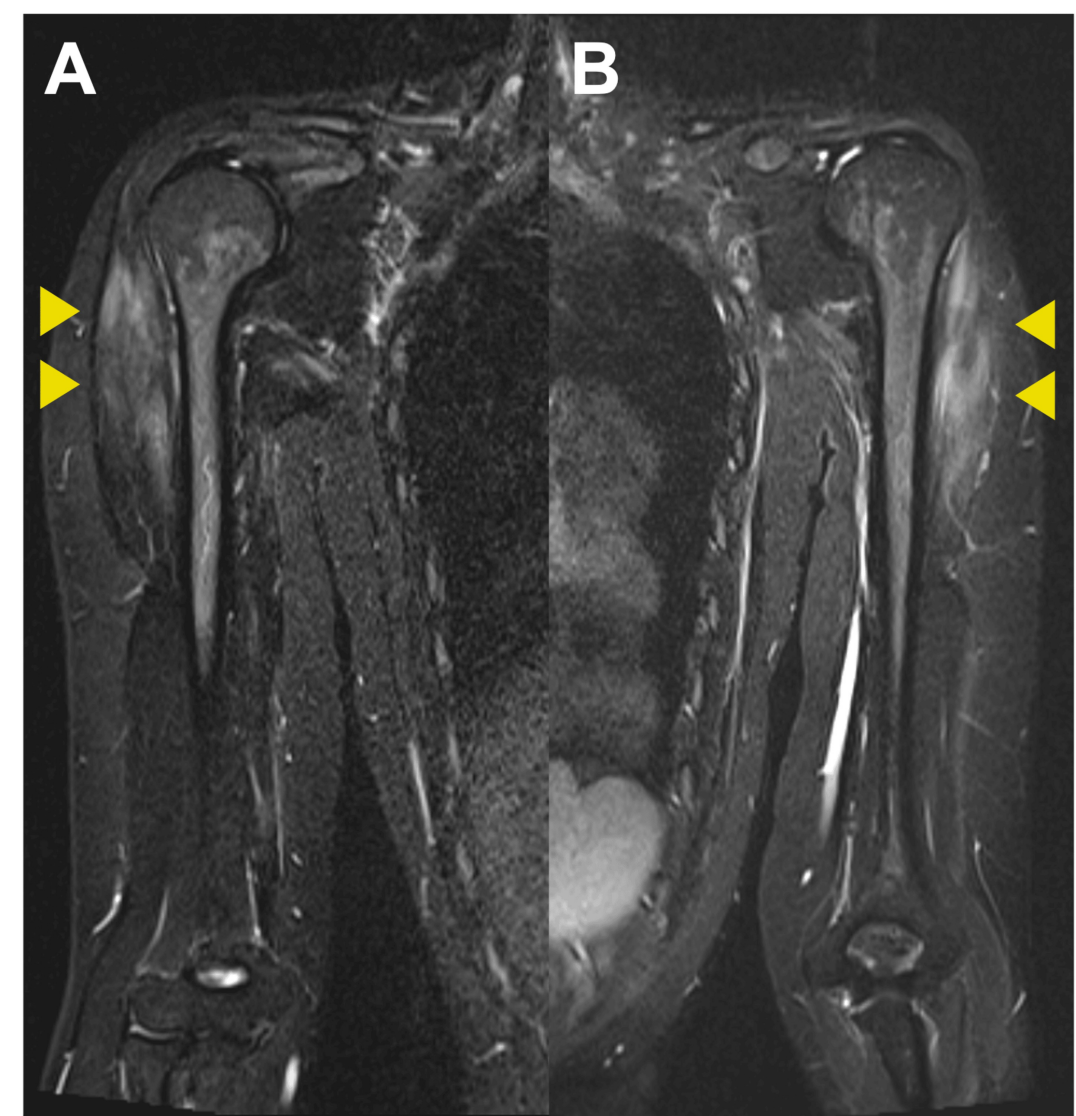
Non-contrast MRI of the patient’s arms. Short tau inversion recovery demonstrated high-intensity lesions in the right (A) and left (B) arm muscles (arrows).
